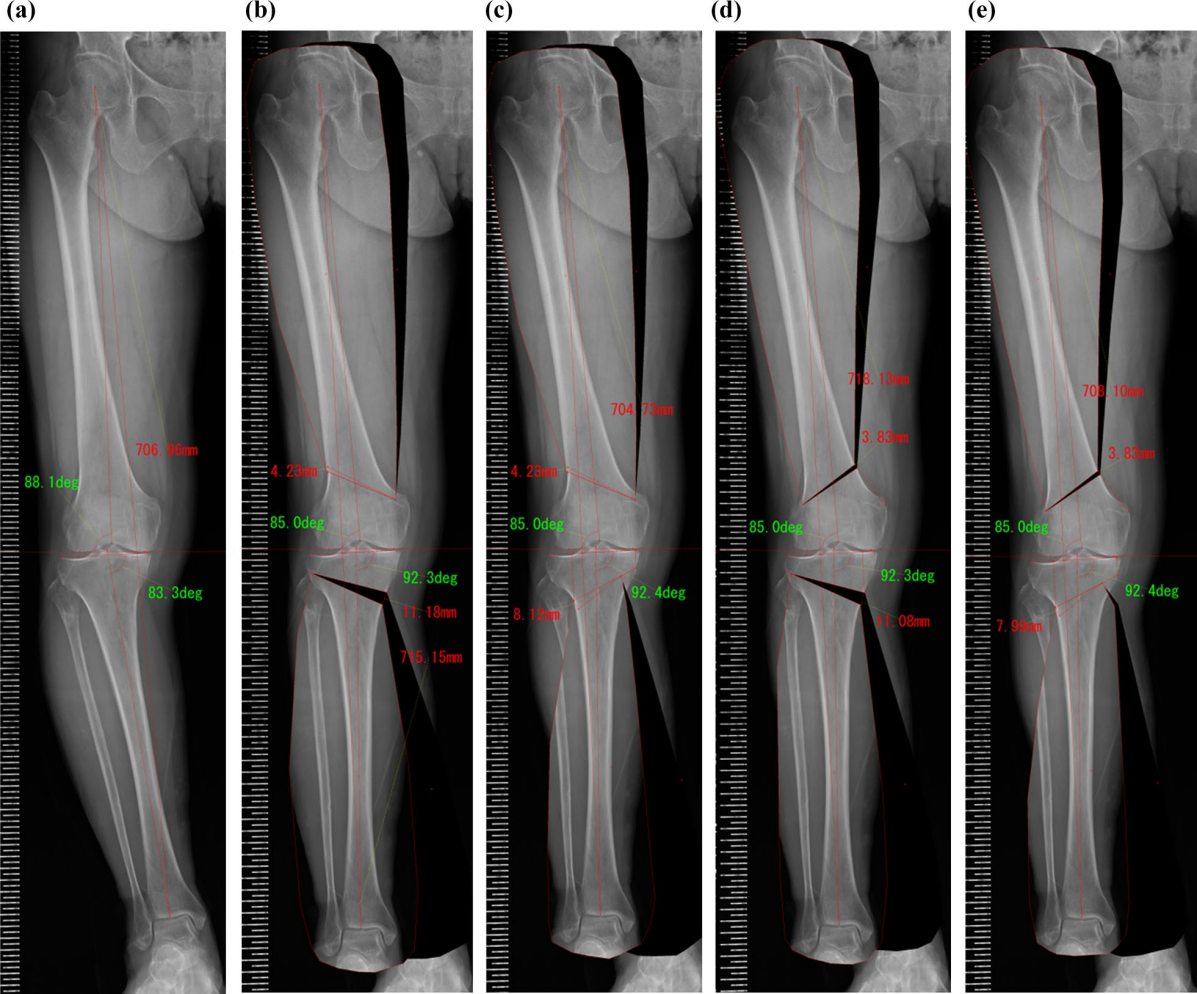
Pre- and post-planning radiographs. a Pre-planning radiograph, b lateral closed wedge distal femoral osteotomy (LCWDFO) + medial open wedge high tibial osteotomy (MOWHTO), c LCWDFO + lateral closed wedge high tibial osteotomy (LCWHTO), d medial open wedge distal femoral osteotomy (MOWDFO) + MOWHTO, and e MOWDFO + LCWHTO
